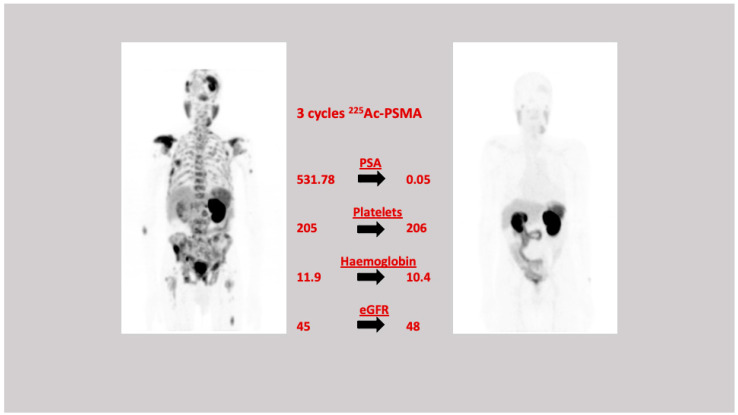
A 79 yr old male with prostate adenocarcinoma. Gleason score = 4 + 3. Previous therapy—prostatectomy. ^68^Ga-PSMA-617 demonstrated widespread axial and appendicular skeletal metastases. He received three cycles of ^225^Ac-PSMA therapy (19 July 2017, 28 September 2017 and 23 November 2017). The patient had an excellent biochemical, clinical, and imaging response and was in remission.
